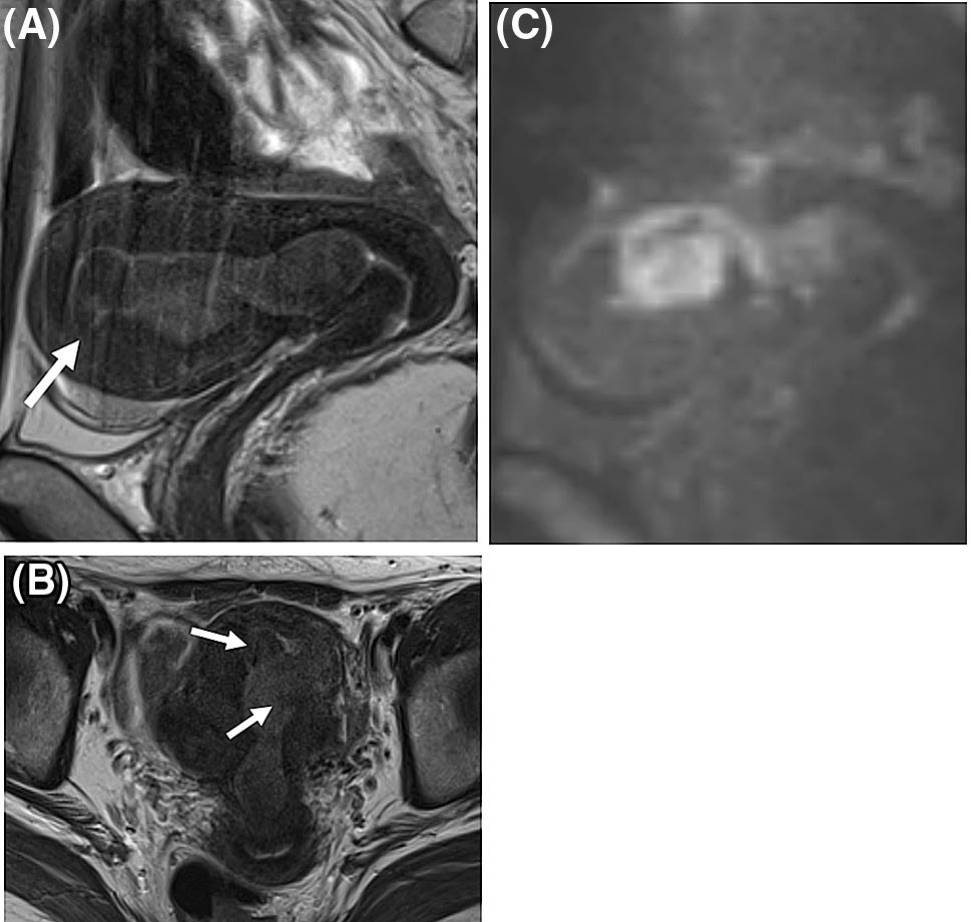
A false-negative case of low-grade endometrial stromal sarcoma. Tumor predominantly located in endometrial cavity with a small portion of myometrial invasion (A, arrow) on T2-weighted images. There might be intra-tumoral low signal intensity (SI) bands on T2-weighted images (B, arrows), but unclear. The tumor showed high SI on diffusion-weighted images (C) with low apparent diffusion coefficient values.
